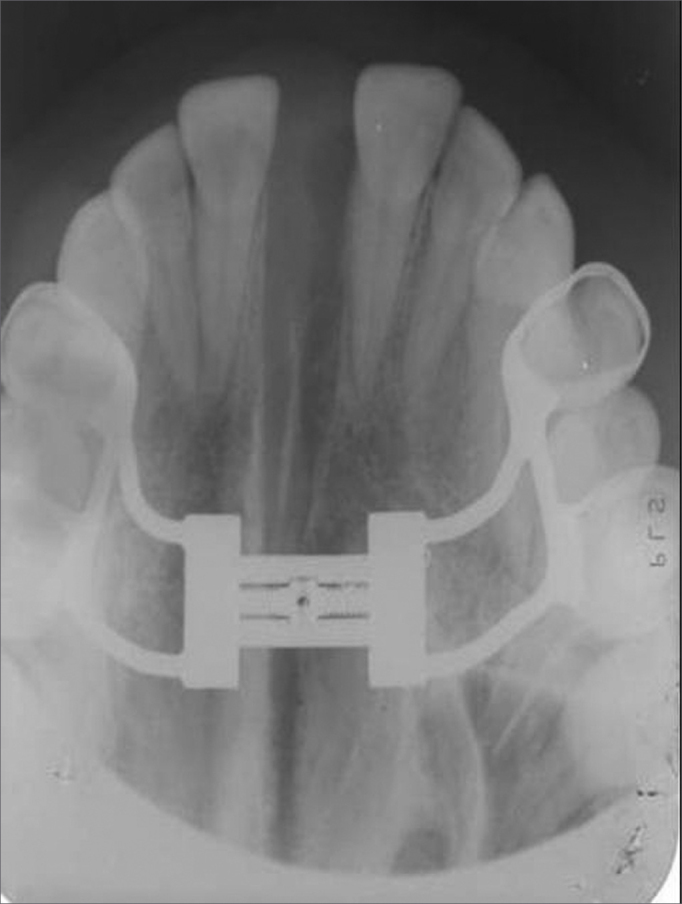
Occlusal radiograph of the maxilla after the maxillary expansion procedure. Note the opening of the midpalatal suture.
